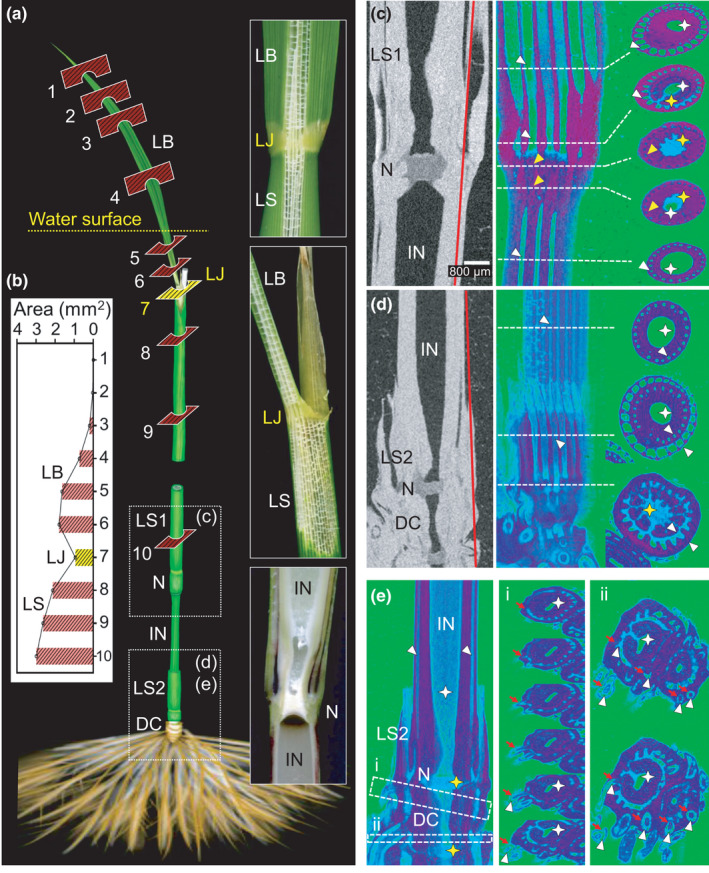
Plant body hollow structures. (a) Anatomy of the hollow structures in the leaf blade (LB), the leaf sheath (LS) including the lamina joint (LJ), and the internode (IN) with a node (N). (b) Area of the aerenchyma in each position indicated in (a). (c–e) Acquisition of two‐dimensional segmentation data for the X‐ray computed tomography (CT) image of a rice plant. The right panel corresponds to the two‐dimensional longitudinal image along the red line in the left panel. The hollow structure (empty or air) is in green, the parenchyma is in red, and the spongy tissue is in blue. White arrowhead, hollow structure in LS and IN; yellow arrowhead, aerenchyma with spongy tissue in the node; white star, hollow structure in IN; yellow star, spongy tissue in the node; red arrows, roots; DC, discrimination center. The scale bar in (c) applies to all X‐ray CT images (c–e).
